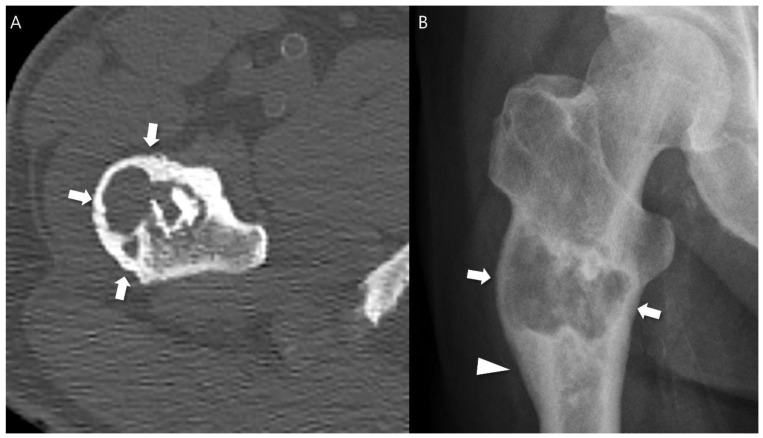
Expansile cortical remodeling in a patient with CS2 in the proximal femur. (A) An axial CT image shows a lobulated mass containing chondroid matrix mineralization and expansile cortical remodeling (arrows). (B) A plain radiograph shows a lobulated mass with expansile cortical remodeling (arrows) with cortical thickening (arrowhead).
